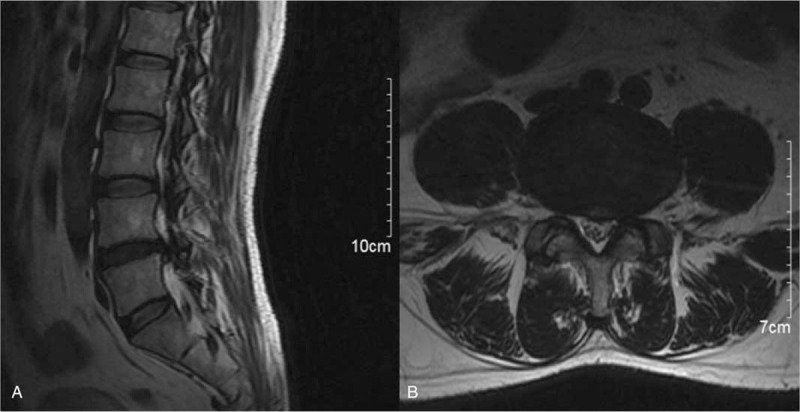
The sagittal (A) and axial (B) of MRI showed LDH (L4-5).
